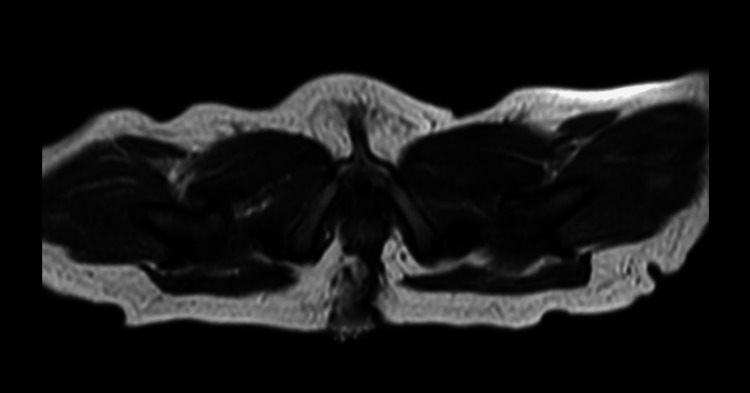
Pelvic MRI showing anomaly presacral with no communication to the rectum on axial view
